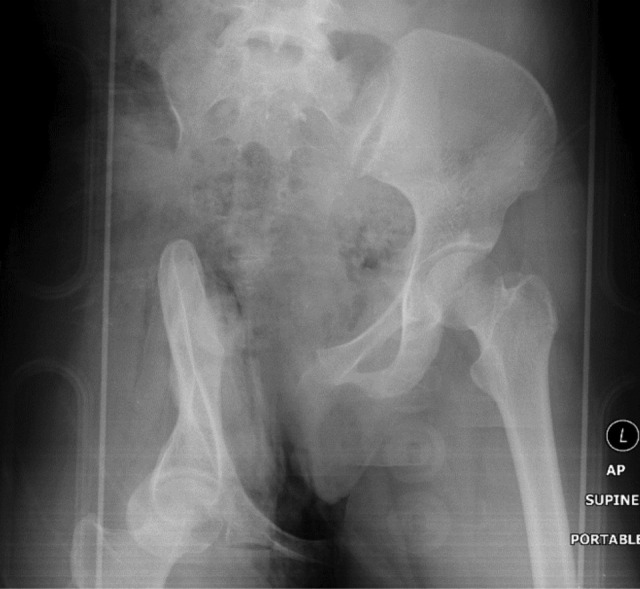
Pelvic anterior-posterior radiograph showed the survivor’s right hemipelvis was sheared inferiorly and malrotated.
